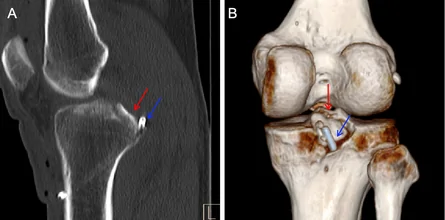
Postoperative (A) CT and (B) 3D reconstruction images of the right knee (red arrows indicate the avulsed bone fragment and blue arrows indicate the EndoButton used for fixation). (3D, 3‐dimensional; CT, computed tomography.)
